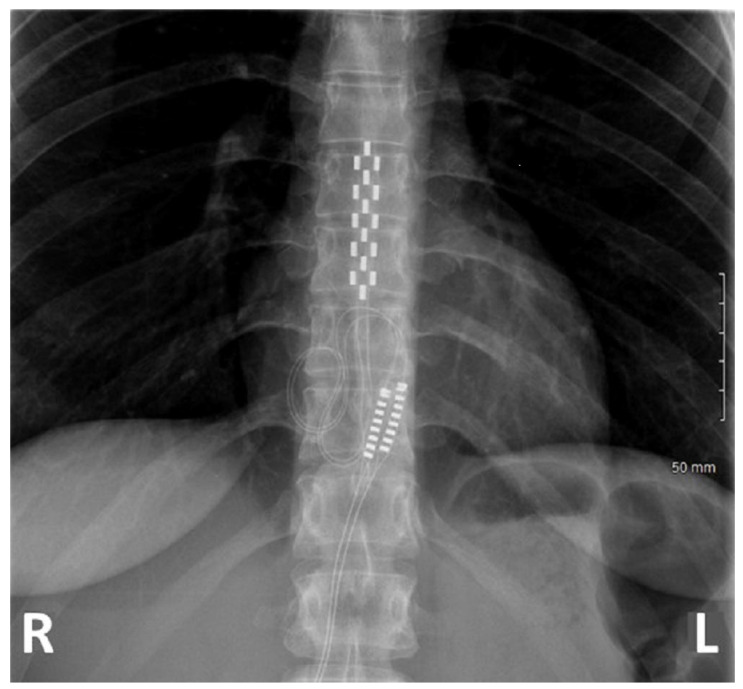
X-ray of a patient implanted at T8-T10 with a multicolumn lead.
